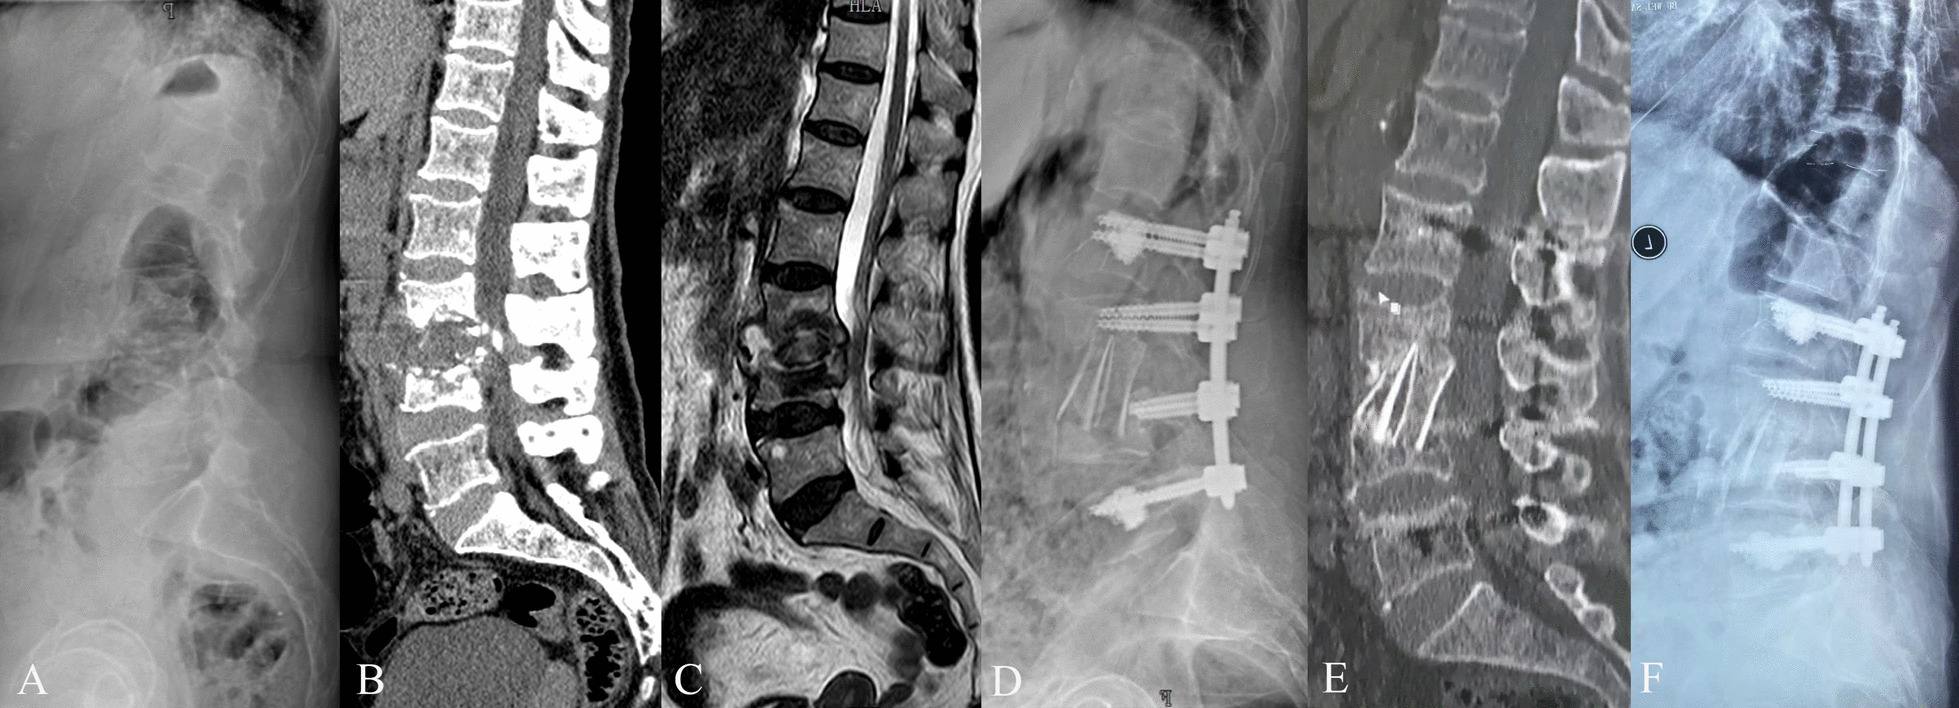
A 81-year-old female with L3-4 tuberculosis and severe osteoporosis. The patient underwent one-stage anterior debridement, bone grafting, and posterior percutaneous fixation with cement-augmented pedicle screws. A–C Preoperative lateral X-ray, sagittal CT scan, and sagittal MRI scan images demonstrated bone destruction and intraspinal abscess. D Postoperative lateral X-ray image. E Sagittal CT scan at 17-month follow-up. F Postoperative X-ray image at 30-month follow-up
